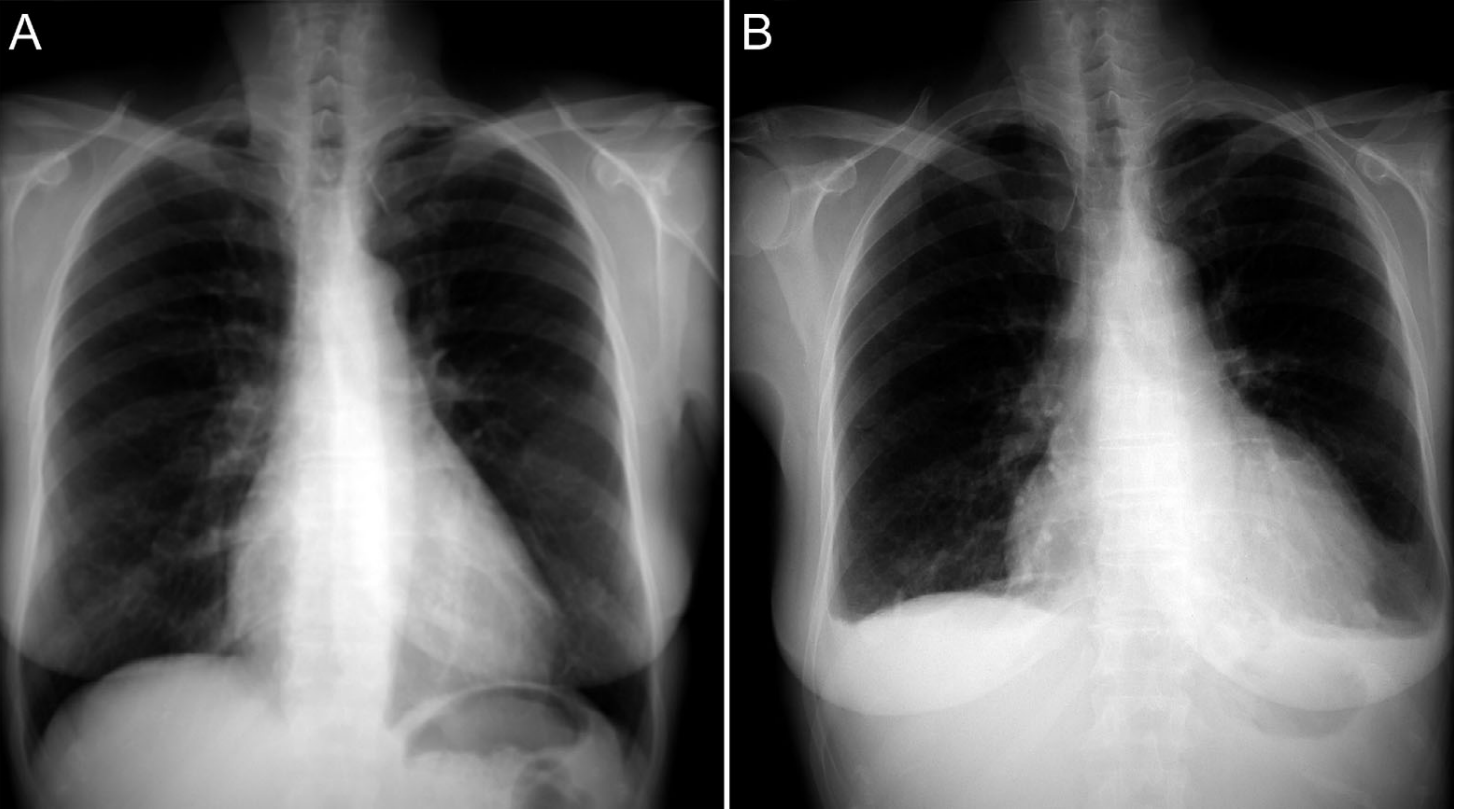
Plain radiographs of the chest (PA views) at admission (a) and four days later (b) with noticeable increase in the cardiac silhouette.
